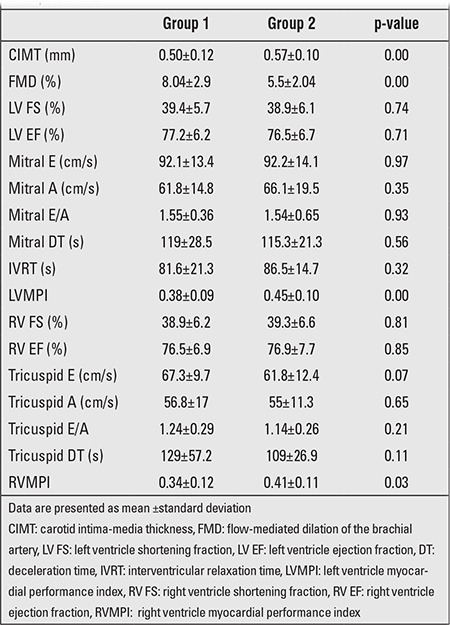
Pulsed-wave Doppler and B-mode echocardiographic data inGroups 1 and 2
